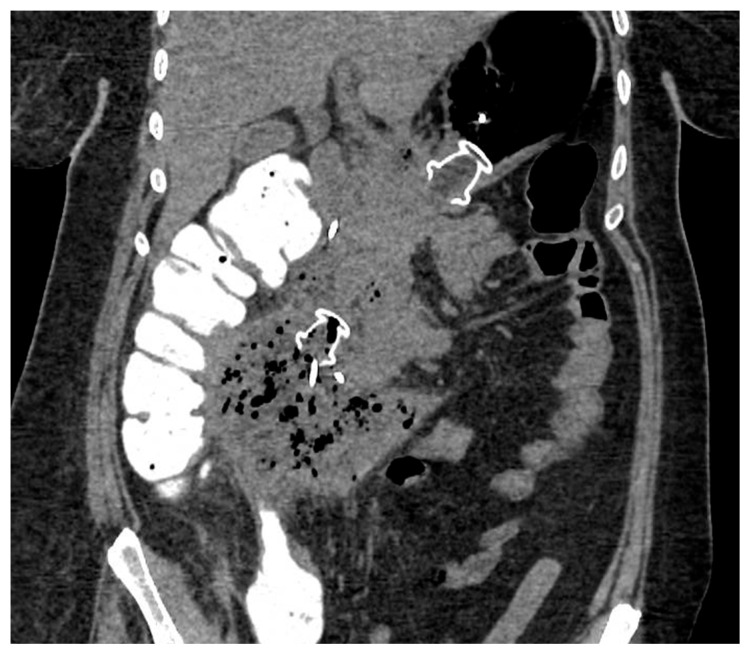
CT scan image of WON drained endoscopically with multiple transluminal gateway technique, with a transgastric 20 mm and a transduodenal 15 mm LAMS. CT: computed tomography; WON: walled-off necrosis; LAMS: lumen-apposing metal stent.
